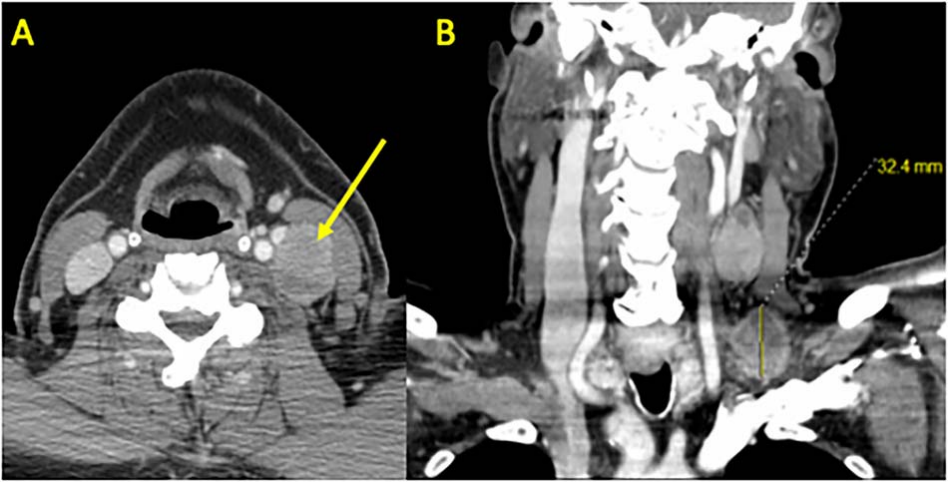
Preoperative CT Scan with Contrast. (A) Axial and (B) coronal sections demonstrating bulky left neck lymphadenopathy. Shown are a 3.2 × 2.8 cm level 4 mass in the supraclavicular fossa and a 3.9 × 2.3 cm level 3 mass adjacent to the internal jugular vein and carotid artery. A left thyroid 1.3 cm nodule was identified (not shown) and confirmed as PTC using fine needle aspiration.
